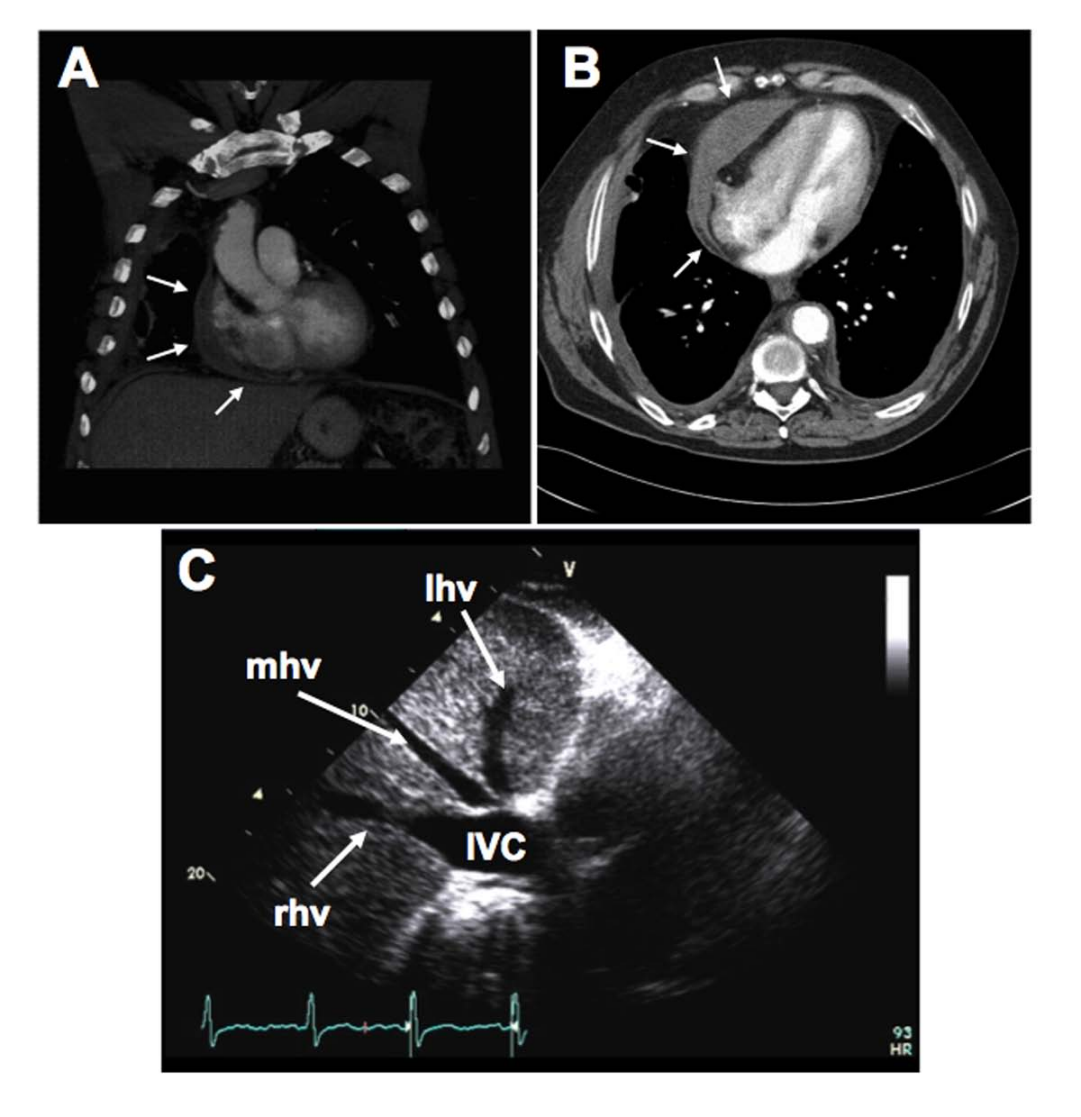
Computed tomography (CT) scan identifying pericardial thickening and echocardiogram showing dilated intrahepatic vein and inferior vena cava: (a,b) CT axial and coronal views of pericardial and pleural thickening. Arrows point to areas of thickened pleura and pericardium. (c) Transthoracic echocardiogram (TTE) showing dilated intrahepatic vein and inferior vena cava (IVC). The terms lhv, mhv, and rhv correspond to left, middle, and right hepatic veins, respectively.
